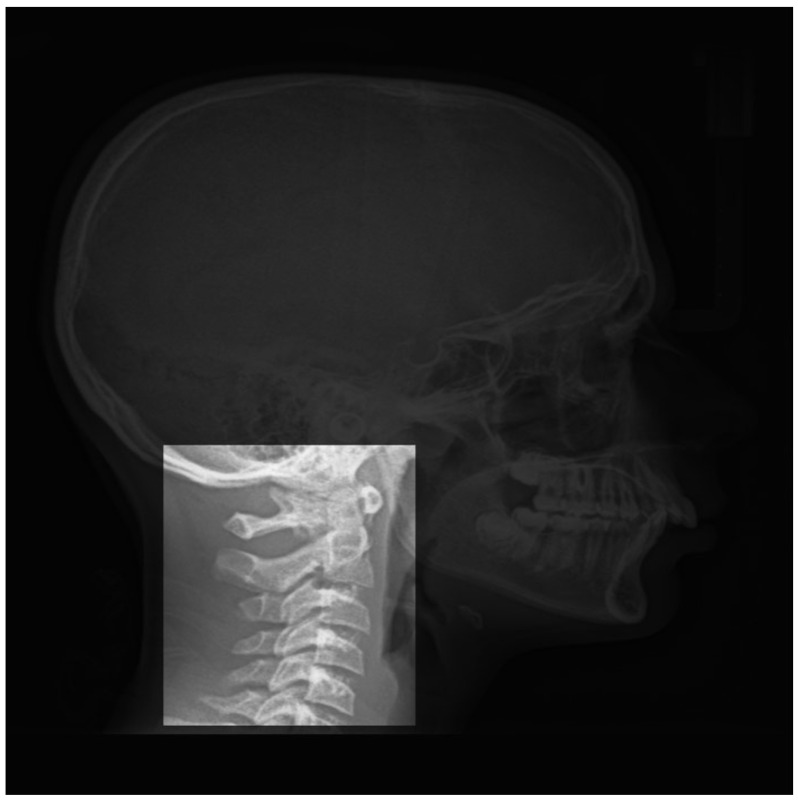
Isolated part (vertebra) of a standard radiography.
